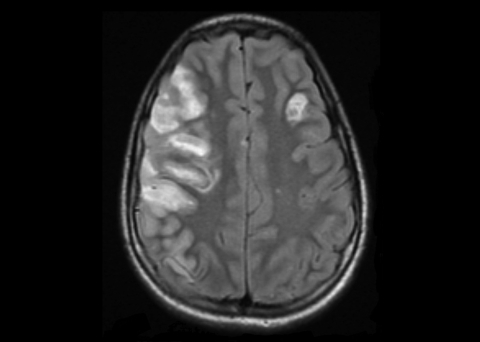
Magnetic resonance image of the head of the patient, a 13-year-old boy, showing multiple areas of infarction bilaterally with the largest in the right middle cerebral artery distribution and a smaller one in the left frontal region consistent with embolic infarcts.
